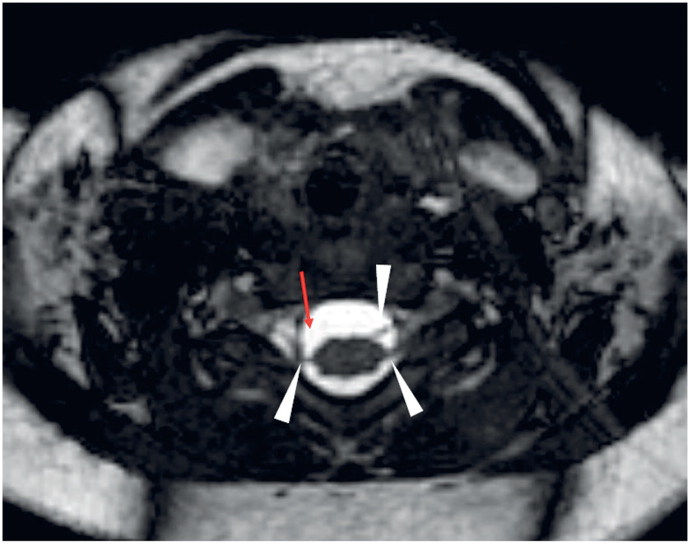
Axial BFFE MR image (0.5 mm) in a 4-month-old girl (patient 26) with brachial plexus birth injury on the right side. Partial avulsion of C8 root: ventral root is avulsed (red arrow), dorsal C8 root is intact (arrowhead). Left C8 nerve roots are normal (arrowheads).
